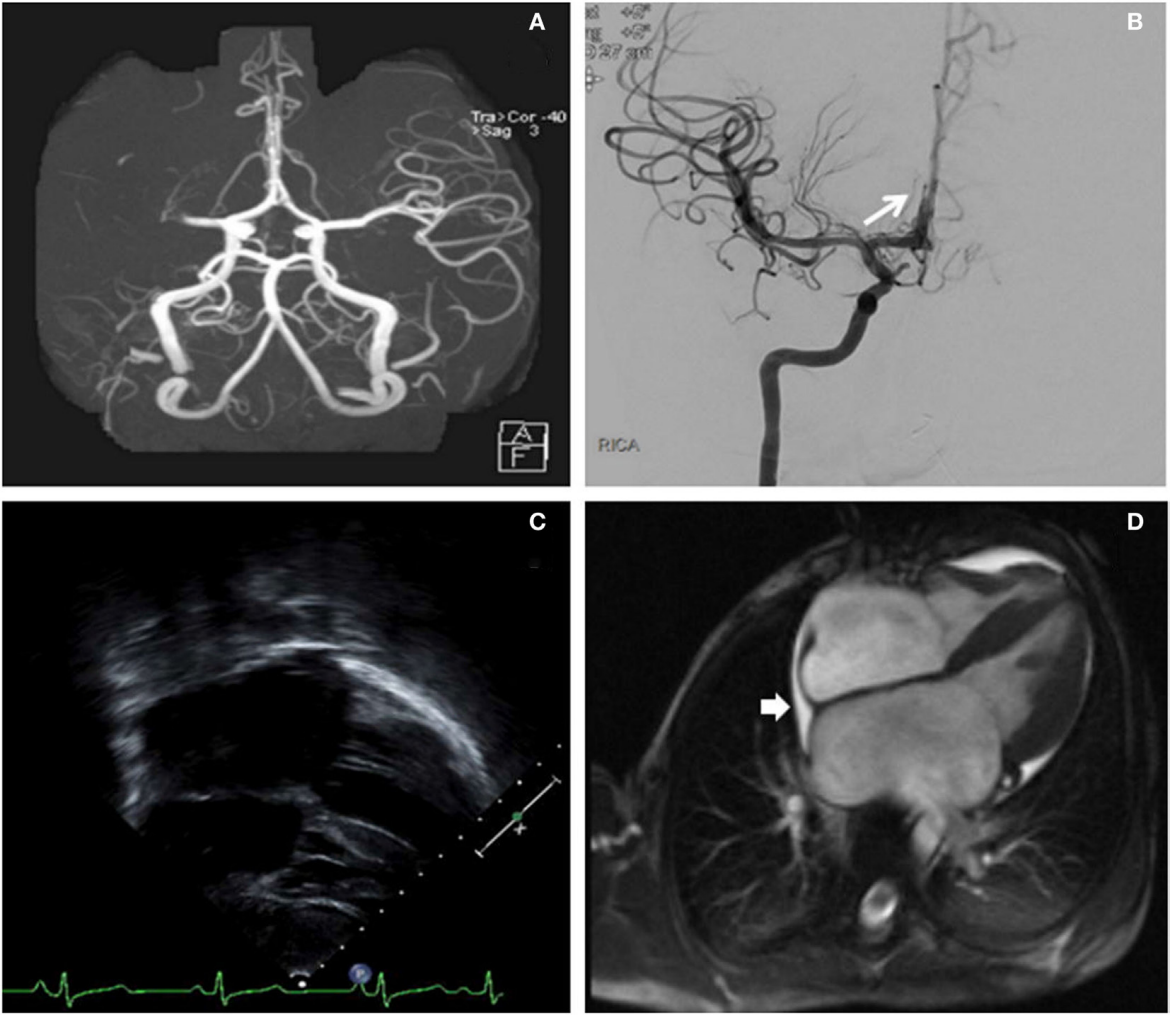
(A) Right middle cerebral artery (MCA) cutoff (M1) in a computed tomography angiogram (linear arrow). (B) Digital subtraction angiography (antero-posterior) exhibiting a recanalization of the right MCA post-thrombectomy. The long arrow identifies the thromboembolic occlusion of a distal right anterior cerebral artery segment. (C) Long-axis echocardiogram demonstrating biatrial enlargement. (D) Cardiac magnetic resonance imaging re-demonstrating the biatrial enlargement, and also revealing a small pericardial effusion (short arrow).
